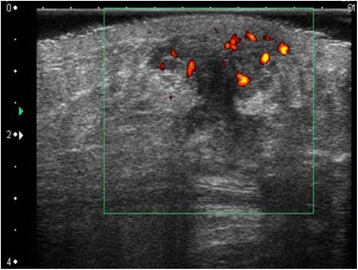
Ultrasonography revealed a hypoechoic lesion 20 mm in size, with border irregularity and blood flow, initially diagnosed malignancy suspected
